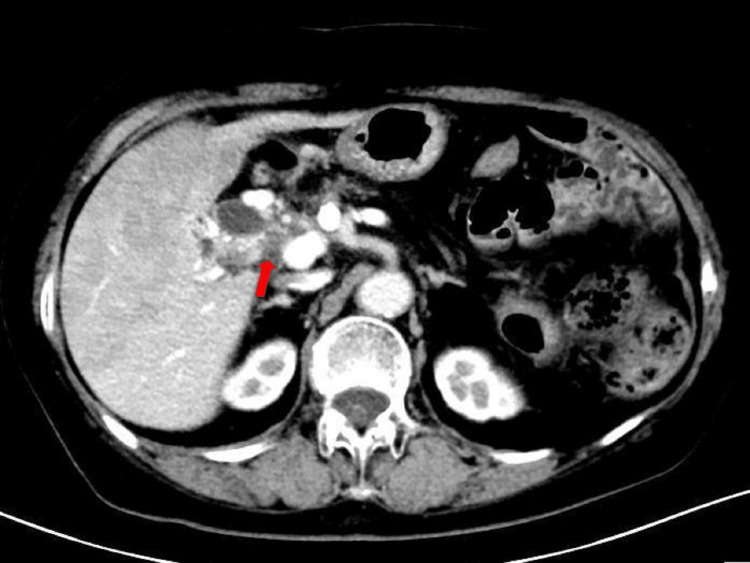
Follow-up CT in July 2024, showing that the tumor continues to decrease (red arrow) CT: computed tomography
